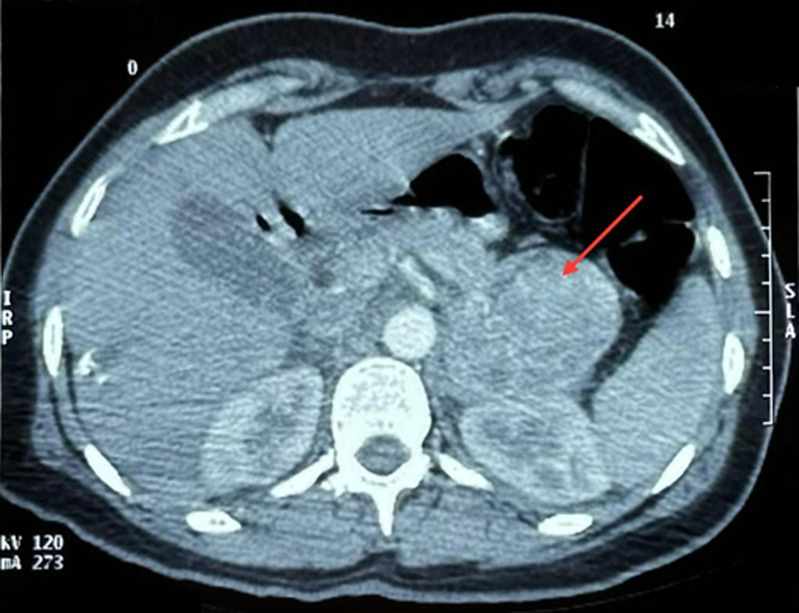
abdominal computed tomography scan revealing left adrenal mass measuring 67 x 54 x 66 mm, suggestive of pheochromocytoma
